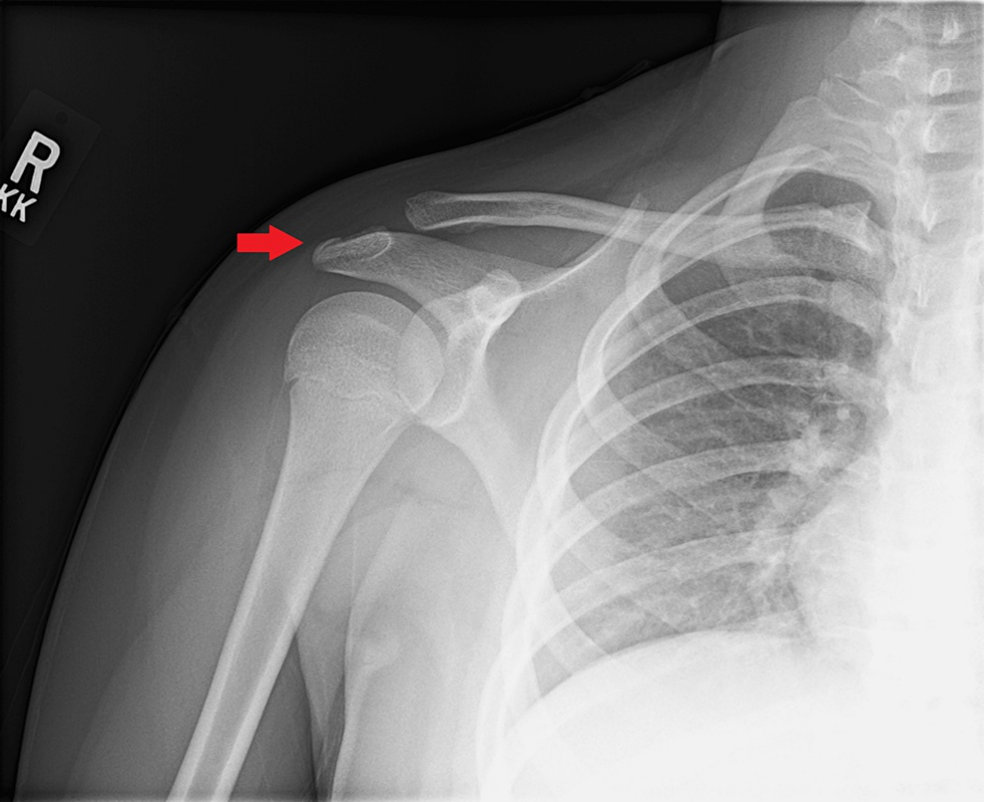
Anterior-posterior radiograph of the right shoulder in external rotation showing a non-angulated and minimally displaced avulsion fracture of the right distal acromion (red arrow)
